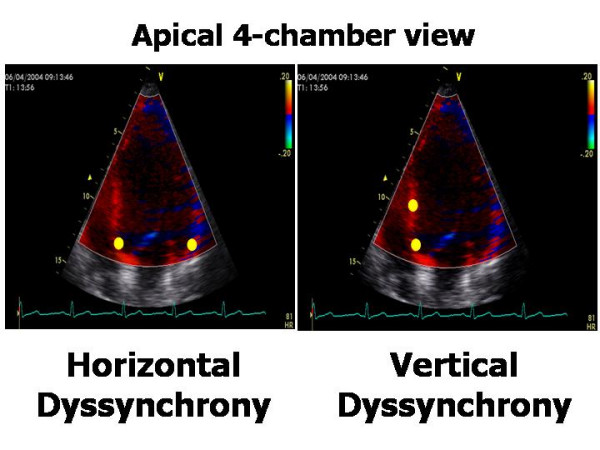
Methodology for recording and measuring intra-ventricular horizontal and vertical dyssynchrony by off-line color Tissue Doppler techniques. Horizontal dyssynchrony occurs between opposite walls. Vertical dyssynchrony is between different segments of the same wall.
